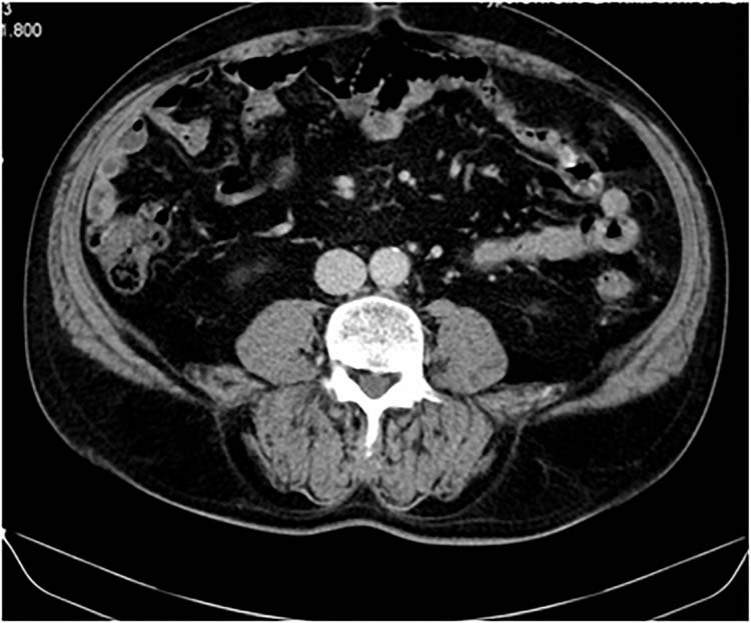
CT image at 1-year follow up showing no local tumor recurrence.
